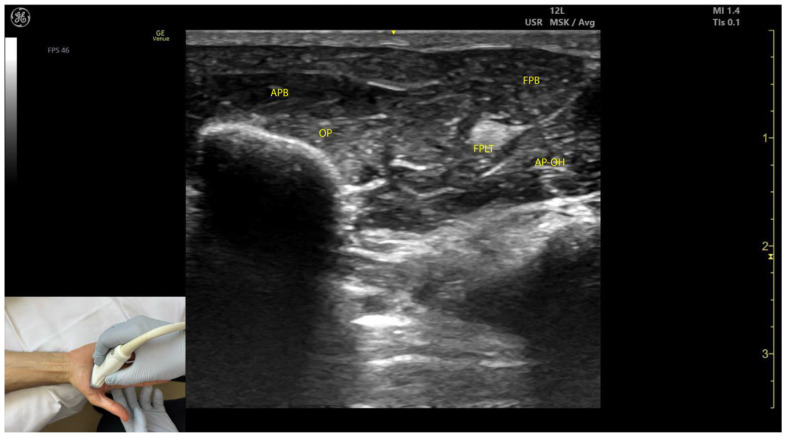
Ultrasound anatomy of the abductor pollicis brevis (APB) with key landmarks: APB—abductor pollicis brevis; OP—opponens pollicis; FPB—flexor pollicis brevis; AP-OH adductor pollicis oblique head; FPLT—flexor pollicis longus tendon.
